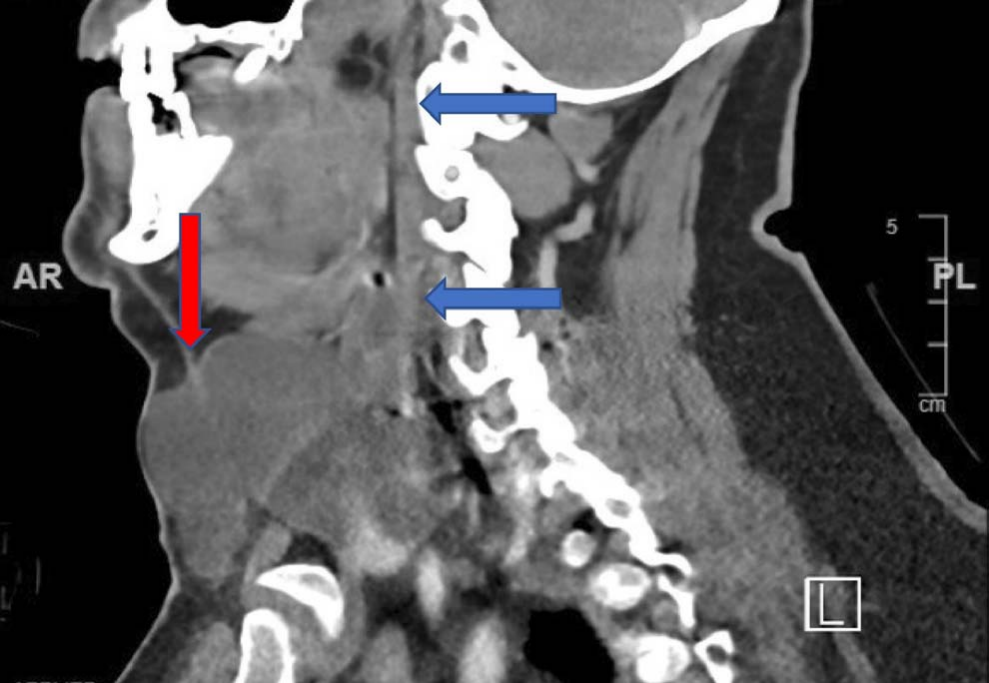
Sagittal computed tomography image of the neck. Blue arrows indicate extensive craniocaudal retropharyngeal cerebrospinal fluid (CSF) collection. Red arrow indicates large CSF collection encompassing the cricoid cartilage with anterior mass effect and tracheal compromise.
